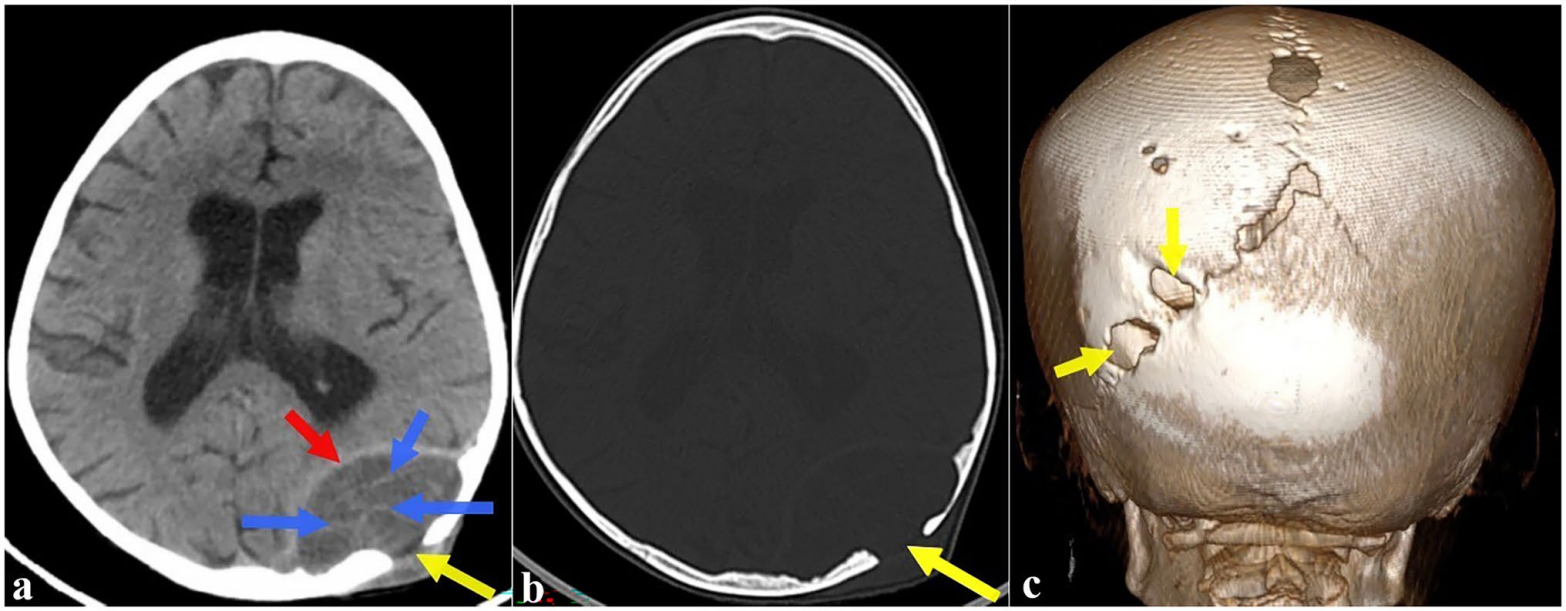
Axial non enhanced brain CT scan in parenchymal window (a), bone window (b) with 3 dimensional reconstruction (c) showing a posterior extra parenchymal left parietal cystic mass (a, red arrow) communicating with the subcutaneous tissues through bone defects (a, b, c, yellow arrows) containing membranes (a, blue arrows)
